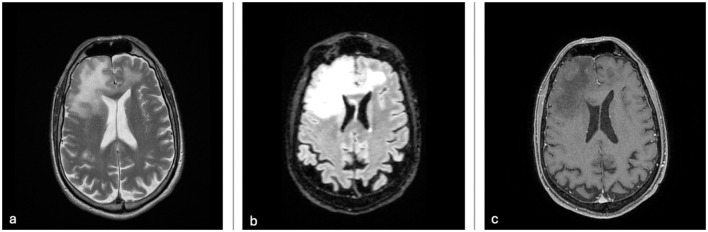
(a) Axial T2-weighted MRI; (b) Axial CUBE-FLAIR MRI; and (c) Axial post-gadolinium 3D-T1 weighted FSPGR MRI. It is possible to appreciate the diffuse pattern of right frontal lobe white matter infiltration with cortical sparing and the complete absence of contrast uptake. The mass effect exerted on the surrounding brain parenchyma is minimal.
